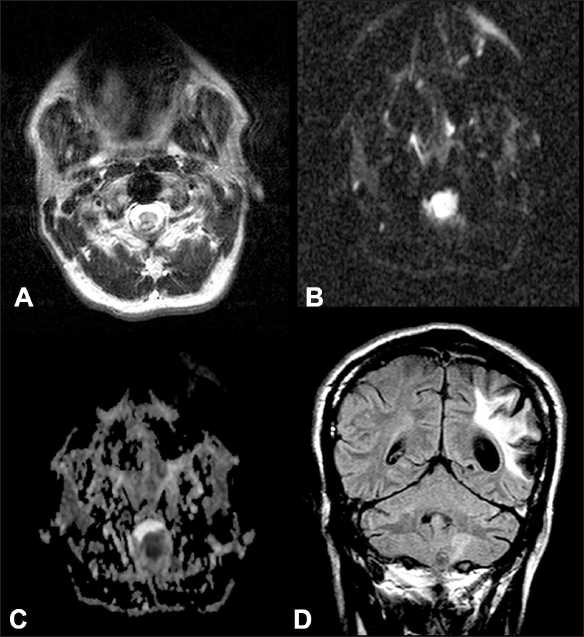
Early MRI evaluation in a 43-year-old man with balance problems and a history of IVDA. T2-weighted axial image at the level of the craniocervical junction (A) with dorsally located hypointensity (arrow) with corresponding true diffusion restriction as seen on diffusion weighted imaging (B), and apparent diffusion coefficient (C) images. Subtle increase in FLAIR signal (D) is noted in the left cerebellar hemisphere (arrowhead). Encephalomalacia from the remote infarct is seen in the left parietal lobe is also seen.
